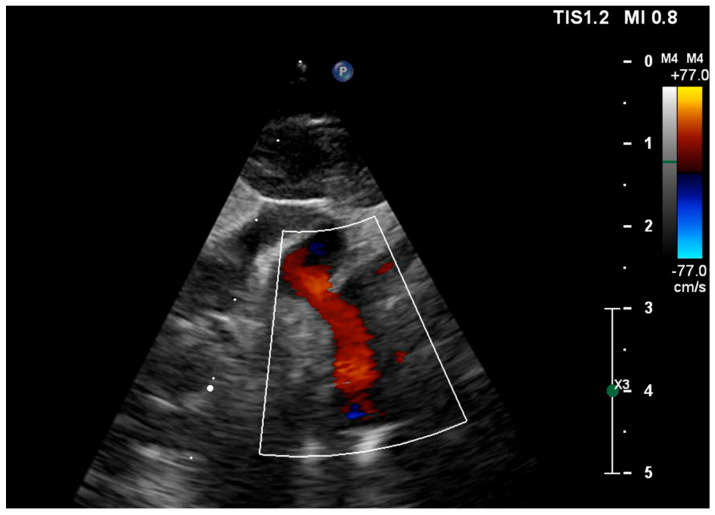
Neonatal echocardiography in the suprasternal view demonstrating retrograde perfusion of the aortic arch.
